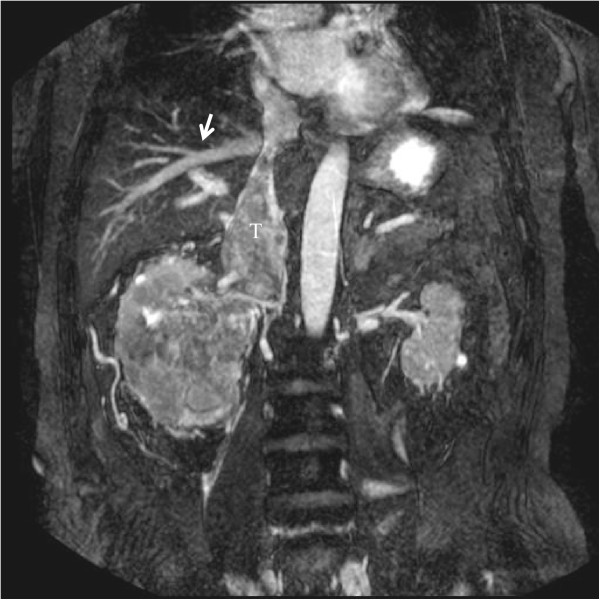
Magnetic resonance images of renal cell carcinoma with tumor thrombus (level III). Magnetic resonance images of a 75-year-old man with right renal cell carcinoma showing a large tumor thrombus (T) extending into the retrohepatic inferior vena cava at the main hepatic vein (white arrow, level III).
